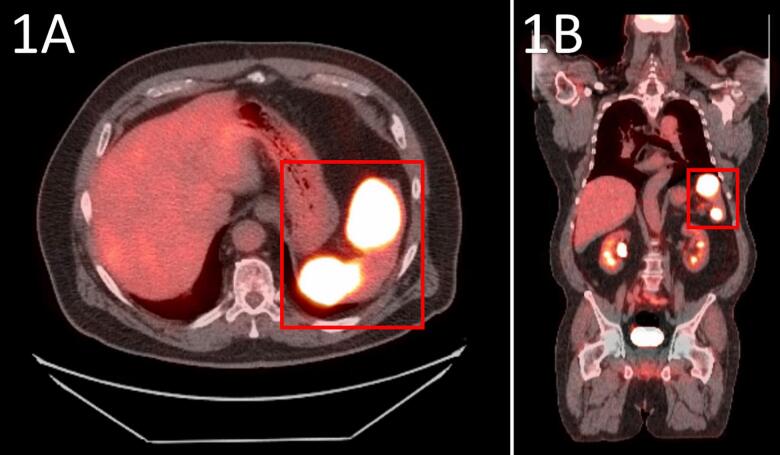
Axial (A) and coronal (B) PET/CT abdomen-pelvis scans demonstrating two hyper-attenuating lesions within the spleen, estimated at 4.1 × 2.5 cm and 1.5 × 3.0 cm. Hyper-attenuating PET scan images demonstrate areas of hypermetabolism, concerning for splenic malignancy, indicating definitive splenectomy and consequent pathology.
